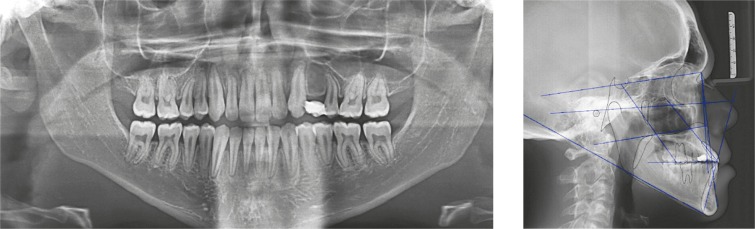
Panoramic radiograph and lateral cephalogram after treatment.
